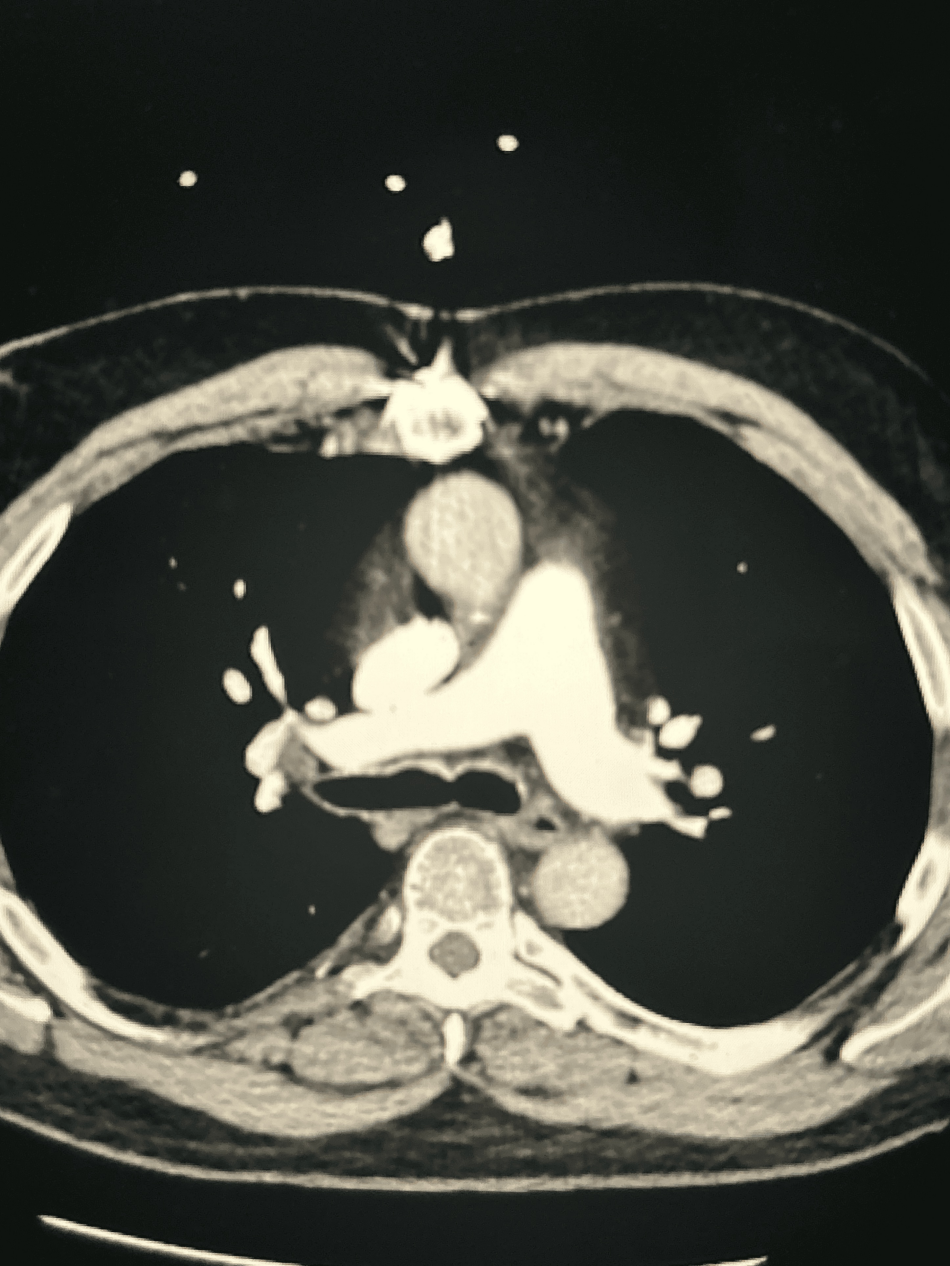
Computed tomography with angiography of the chest demonstrating acute right segmental pulmonary embolus
